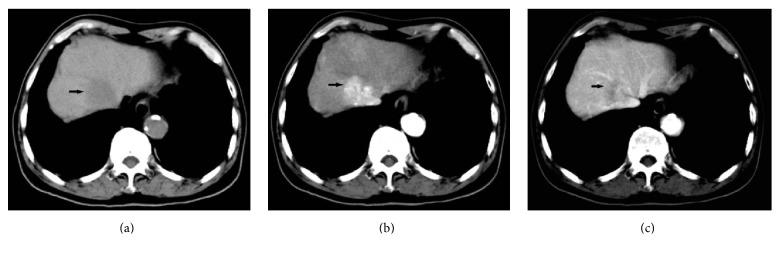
A 48-year-old male patient of G1 with HCC lesions was found liver occupied by ultrasound in physical examination. Unenhanced CT scans (a) revealed a liver S7 low-density round mass with clear boundary. Enhanced CT scans revealed significantly enhanced heterogeneous mass during HAP (b), the density of which was higher than the liver parenchyma of the same slice, and TLC value was 38 HU; the mass density in PVP (c) was lower than liver parenchyma and was enhanced during HAP and hypodense during PVP, and TLC value was −12 HU.
